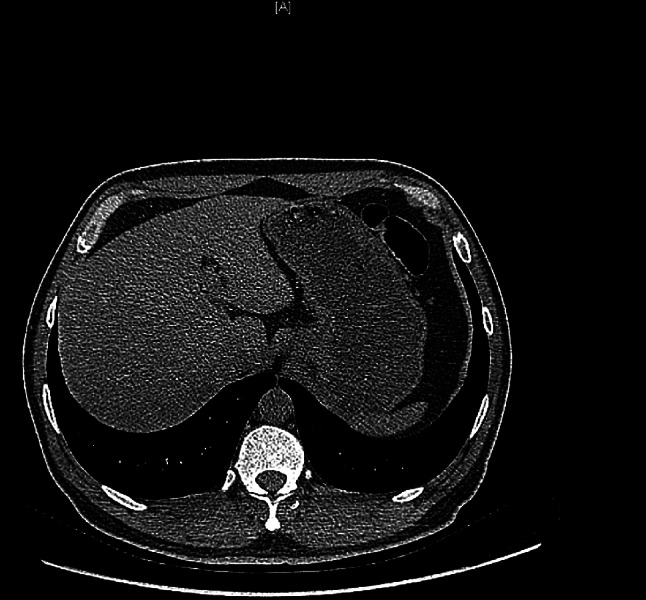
Computed Tomography of upper abdomen. CT showed dilated esophagus and insignificant thickening of the lateral wall of the cardia, beyond that there isn’t any other abnormality
